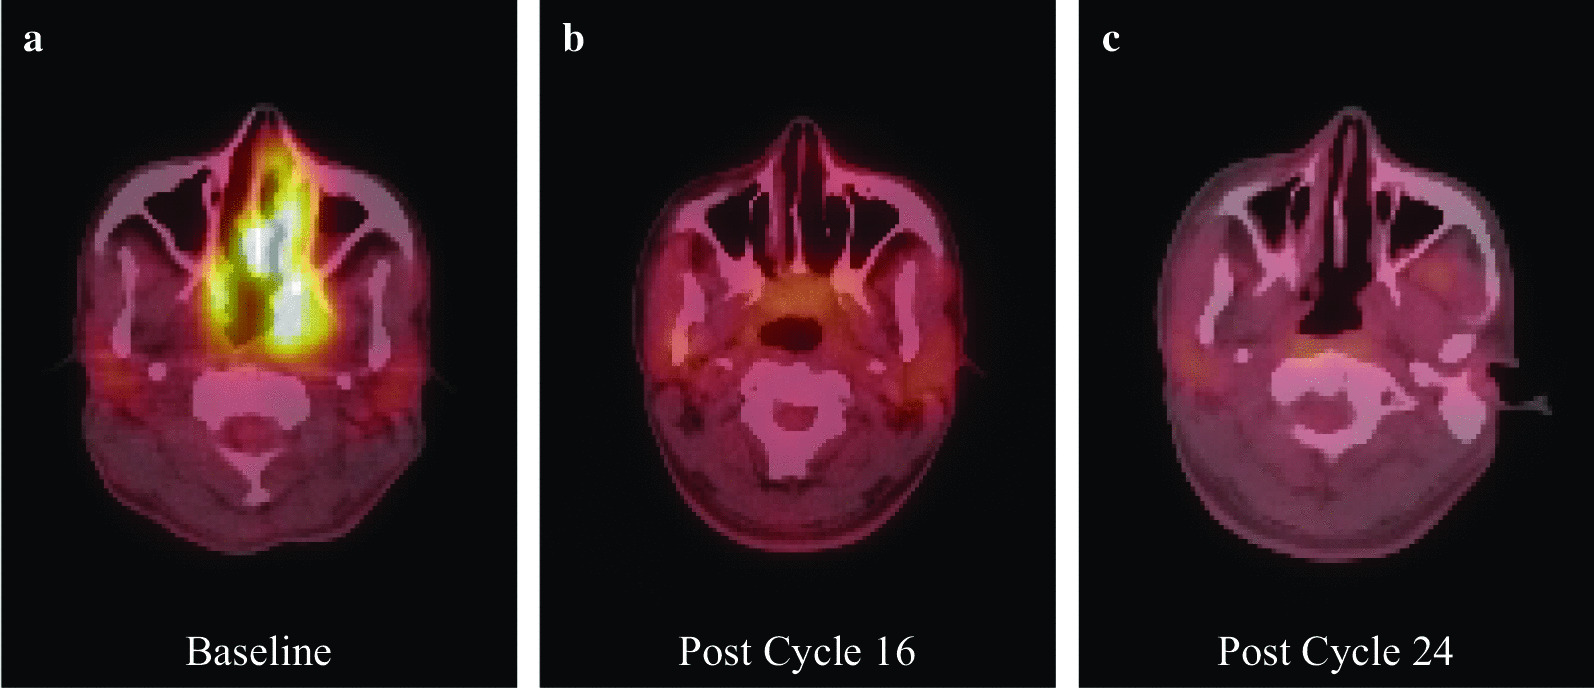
Positron emission tomography-computed tomography (PET-CT) scan at diagnosis of first relapse and after atezolizumab treatment. a Avid left cervical lymphadenopathy and nasopharynx, oropharynx, and soft palate at baseline. b After 16 doses of 1200 mg intravenous infusion of atezolizumab every 3 weeks, showing complete response of the target lesion and overall partial response. c After 24 doses of atezolizumab, the avid region was biopsied and confirmed by pathology to be negative for lymphoma
